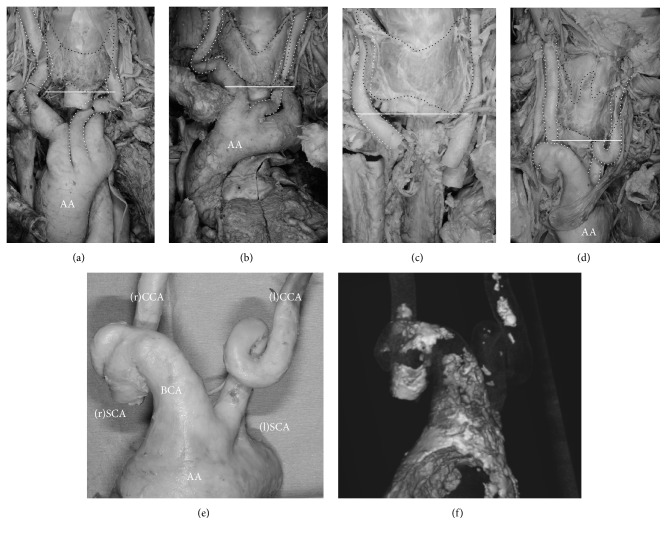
Four cases of tortuous common carotid artery (CCA) reported in the present study. Black dotted lines indicate the thyroid glands. White dotted lines indicate the common carotid arteries. White lines show the inferior border of the thyroid glands. (a) Case 1. Bilateral tortuous CCAs ascend posterior to the thyroid gland. The left side is especially tortuous. (b) Case 2. Bilateral tortuous CCAs ascend posterior to the thyroid gland. The right side is especially tortuous. (c) Case 3. Tortuous CCA on the right side ascends posterior to the thyroid gland. The aortic arch had been removed during dissection. (d) Case 4. Bilateral tortuous CCAs ascend posterior to the thyroid gland with a 360° turn. The brachiocephalic trunk is also tortuous and hypertrophied. (e) Case 4. Aortic arch and its branches after removal from the body. The vascular wall is highly calcified and has lost its elasticity. (f) Case 4. Computed tomography (CT) image shows the calcified part of the aortic arch and its branches. White parts, which had high CT values, are suspected to be calcified. AA: aortic arch; BCA: brachiocephalic artery; CCA: common carotid artery; SCA: subclavian artery.
